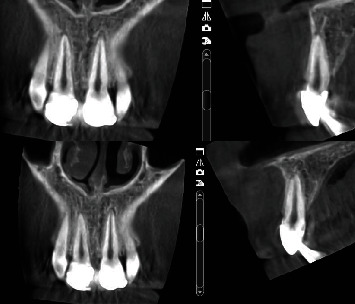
CBCT 5 years after trauma with a normal appearance.
